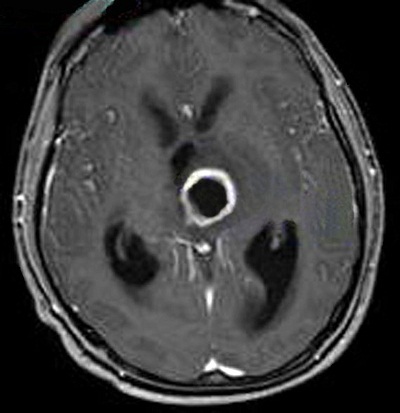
T1-weighted axial MR image with contrast showing a thalamic ring-enhancing lesion in the left side of the brain
